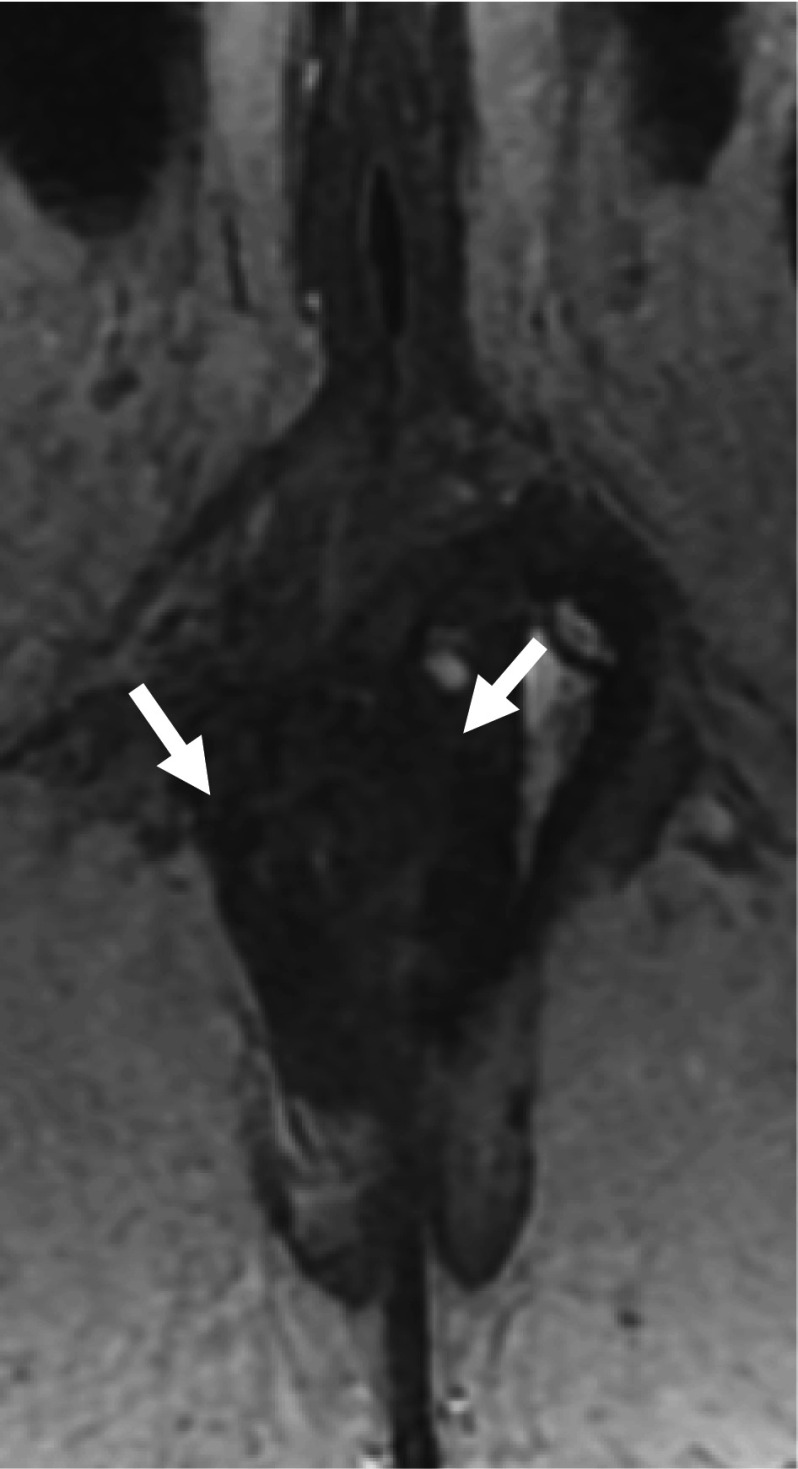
T2-weighted MRI images showed unclear continuity of the anal sphincter muscle on the ventral side. Arrows indicate the edge where the anal sphincter can be firmly recognized
